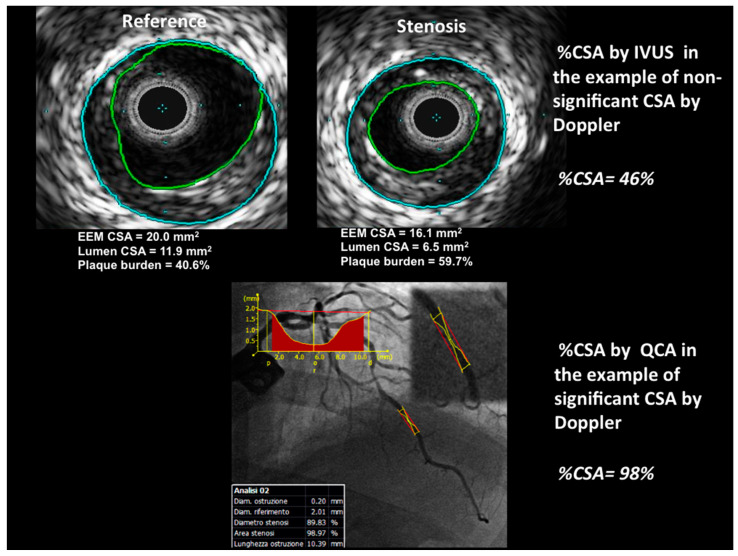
Examples of Doppler stenotic lesions obtained by IVUS (top) and QCA (bottom). The top image shows the same case as that presented in Figure 1 but evaluated by IVUS: the %CSA measured by IVUS (reference lumen area — stenotic lumen area/reference lumen area × 100) gave a %CSA = 46% equal to that of Doppler CSA; the external elastic layer is also traced. The bottom image shows the QCA evaluation of the same stenosis as that assessed in Figure 2 by Doppler. QCA allowed the automatic tracing of the intimal border and the assessment of the minimal (in red) and reference diameters. The %CSA measurements provided a %CSA of 98% confirming the data of the Doppler study. QCA = quantitative coronary angiography; IVUS = intravascular ultrasounds; CSA = cross-sectional area.
